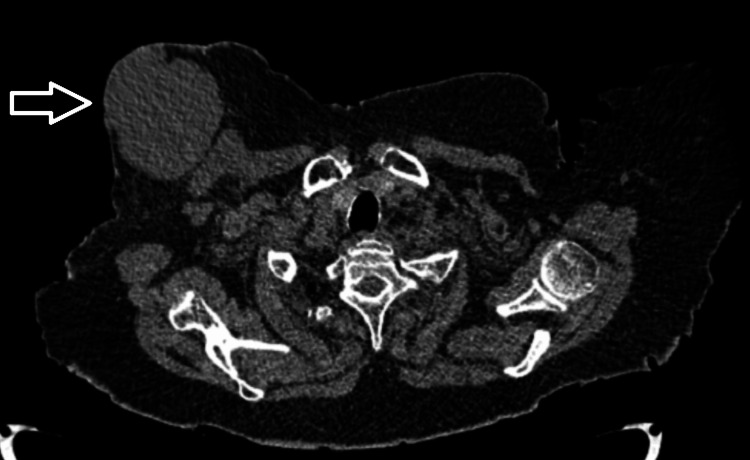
CT scan showing the right breast mass (arrow)
